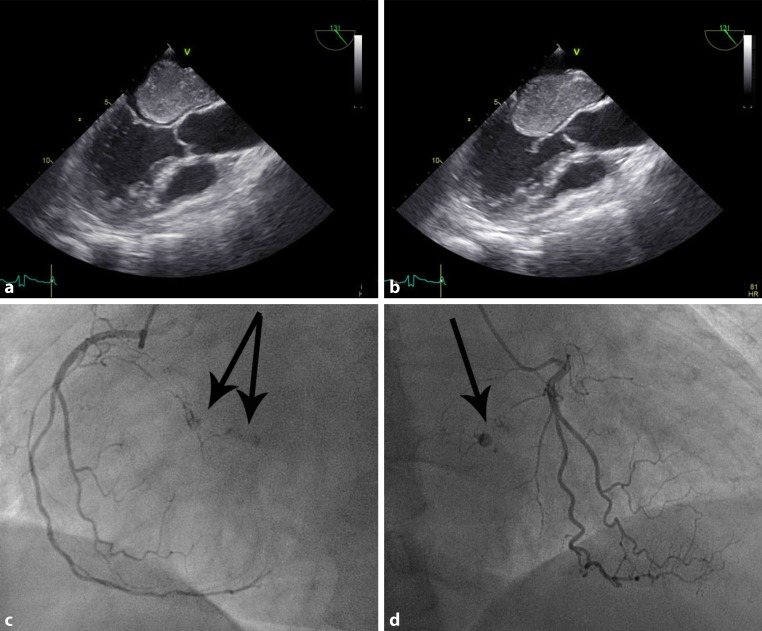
Transoesophageal echocardiographic imaging of left atrial myxoma a in systole and b in diastole, with prolapse of the tumour across the mitral valve. Coronary angiography of the right coronary artery (c, d) showing neovascularisation with a tumour blush (*arrows*)
